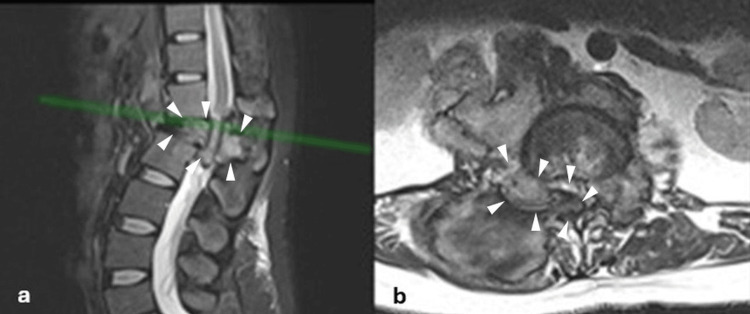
Sagittal and axial magnetic resonance imaging (MRI) of the lumbar spine at the initial encounter a) Sagittal T2-weighted MRI showing a space-occupying vertebral body lesion at the L1 level (arrowheads) with vertebral body collapse and thecal sac compression, with a 45° regional kyphosis. The green reference line indicates the axial slice level displayed in panel b; b) Corresponding axial T2-weighted MRI at the same level demonstrates the intracanal lesion (arrowheads), resulting in marked narrowing of the spinal canal and compression of the adjacent neural structures.
